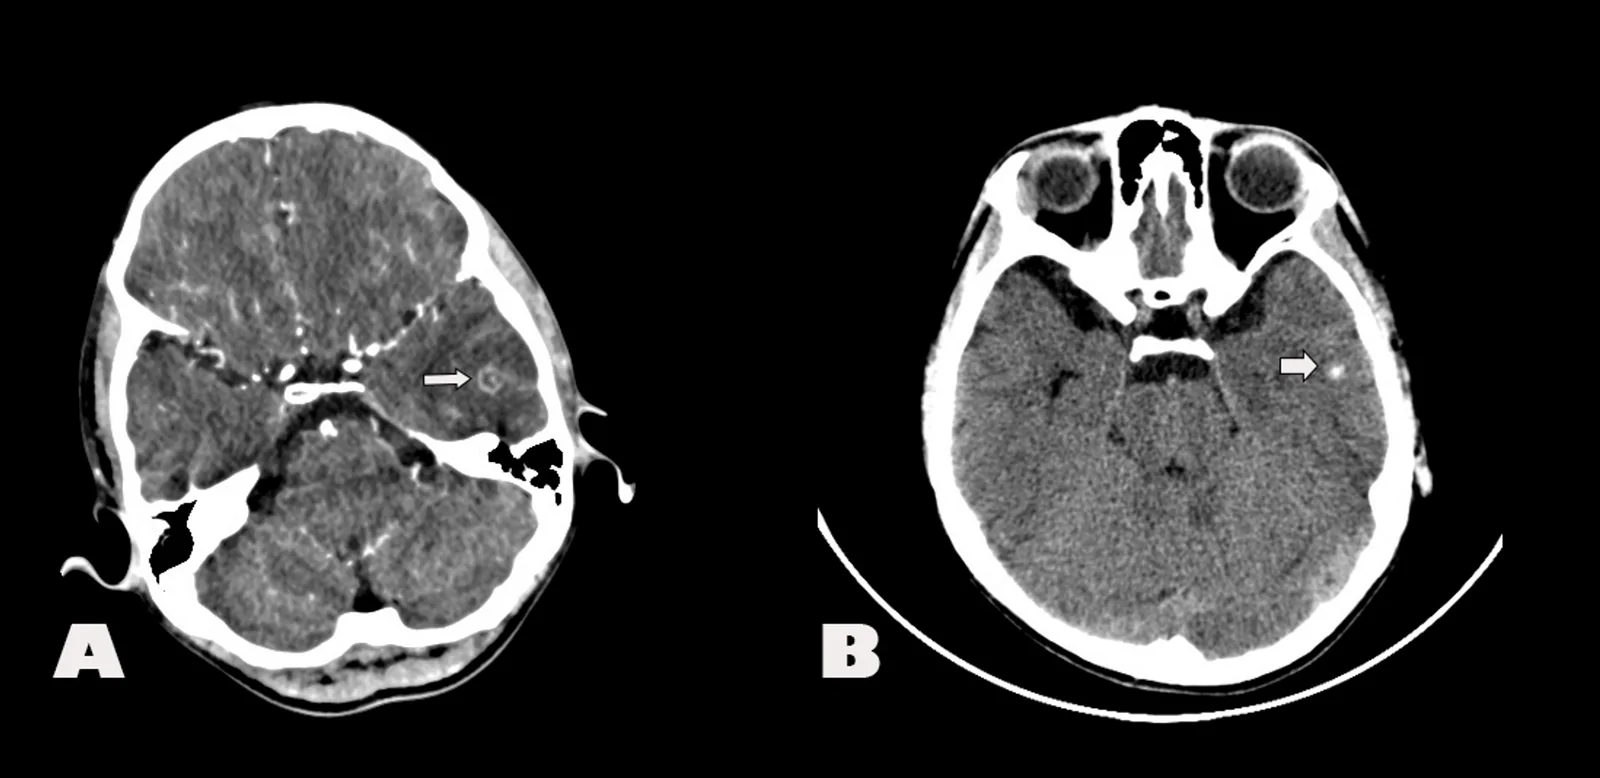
Brain CT scan of a nine-year-old boy showing a ring-enhancing cyst with a scolex inside, located in the temporal lobe (A), and scarring with calcification three months after albendazole treatment (B)
